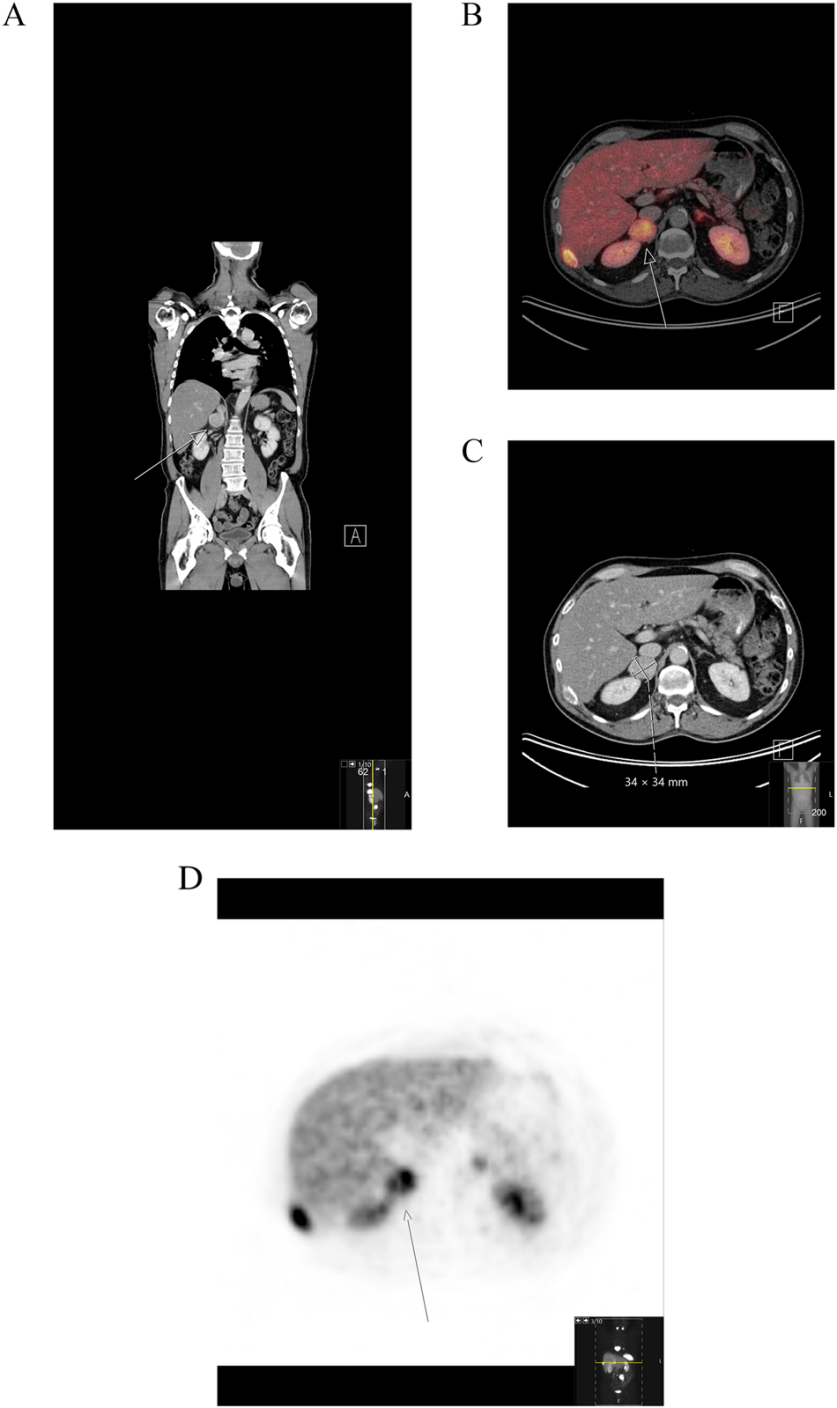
A right adrenal mass at ^68^Gallium-DOTATOC-PET/CT in a middle-aged male. CT image coronar projection (left A), fused CT (middle B), CT image (C) and maximum intensity projection (MIP) projection (D left) of the corresponding axial projection over the adrenal glands. The right adrenal (white arrow) is enlarged and demonstrates a diffuse distinct DOTATOC uptake, not seen in the left, normal-sized adrenal
